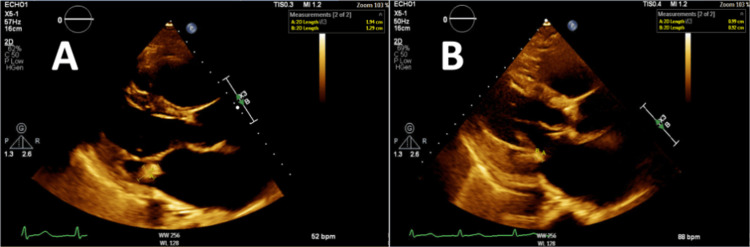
Transthoracic echocardiogram findings. (A) Parasternal long-axis view showing a 1.94 × 1.29 cm vegetation (arrows) on the mitral valve. (B) Parasternal long-axis view with the use of doxycycline and hydroxychloroquine demonstrating a decrease in the size of vegetation to 0.99 × 0.92 cm (arrows).
